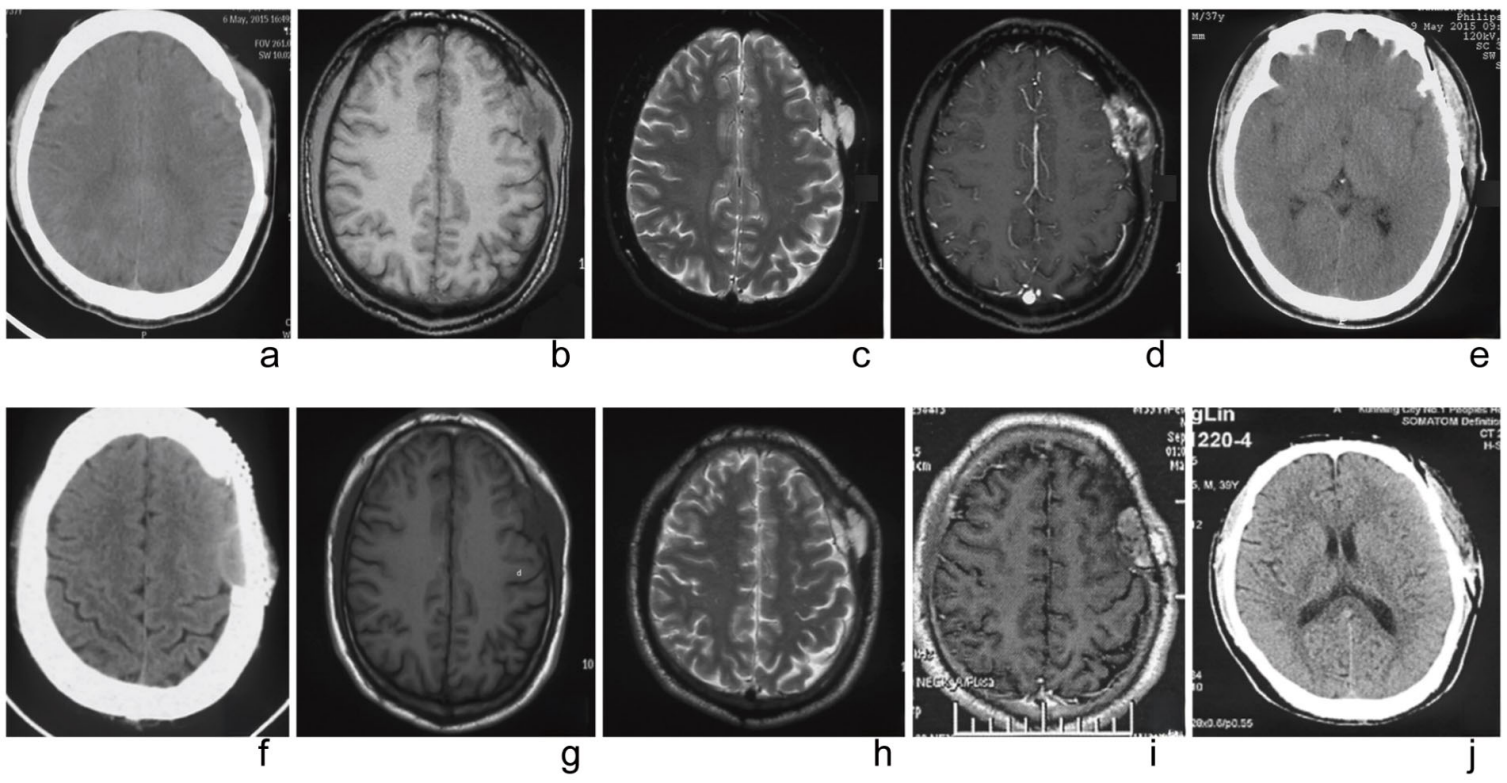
The imaging findings before and after the first surgery. (a) Preoperative CT showed local cranial bone with moth-eaten changes and a spindle-shaped to hypodense shadow on the lateral side of the skull, with uneven density inside. (b) Preoperative MRI (T1WI) displays an uneven signal density. (c) Preoperative MRI (T2WI) appears as an isointense to hyperintense signal. (d) Preoperative MRI enhancement shows ring-like enhancement. (e) A one-week postoperative follow-up cranial CT indicates complete tumor resection. The imaging findings before and after the second surgery. (f) Preoperative CT reveals a low-density lesion with a wide base and clear boundary. (g) Preoperative MRI (T1WI) shows the soft tissue as a lower-density shadow. (h) Preoperative MRI (T2WI) presents as an isointense to hyperintense signal. (i) Preoperative MRI enhancement demonstrates uneven enhancement. (j) A one-week postoperative follow-up cranial CT indicates complete tumor resection.
